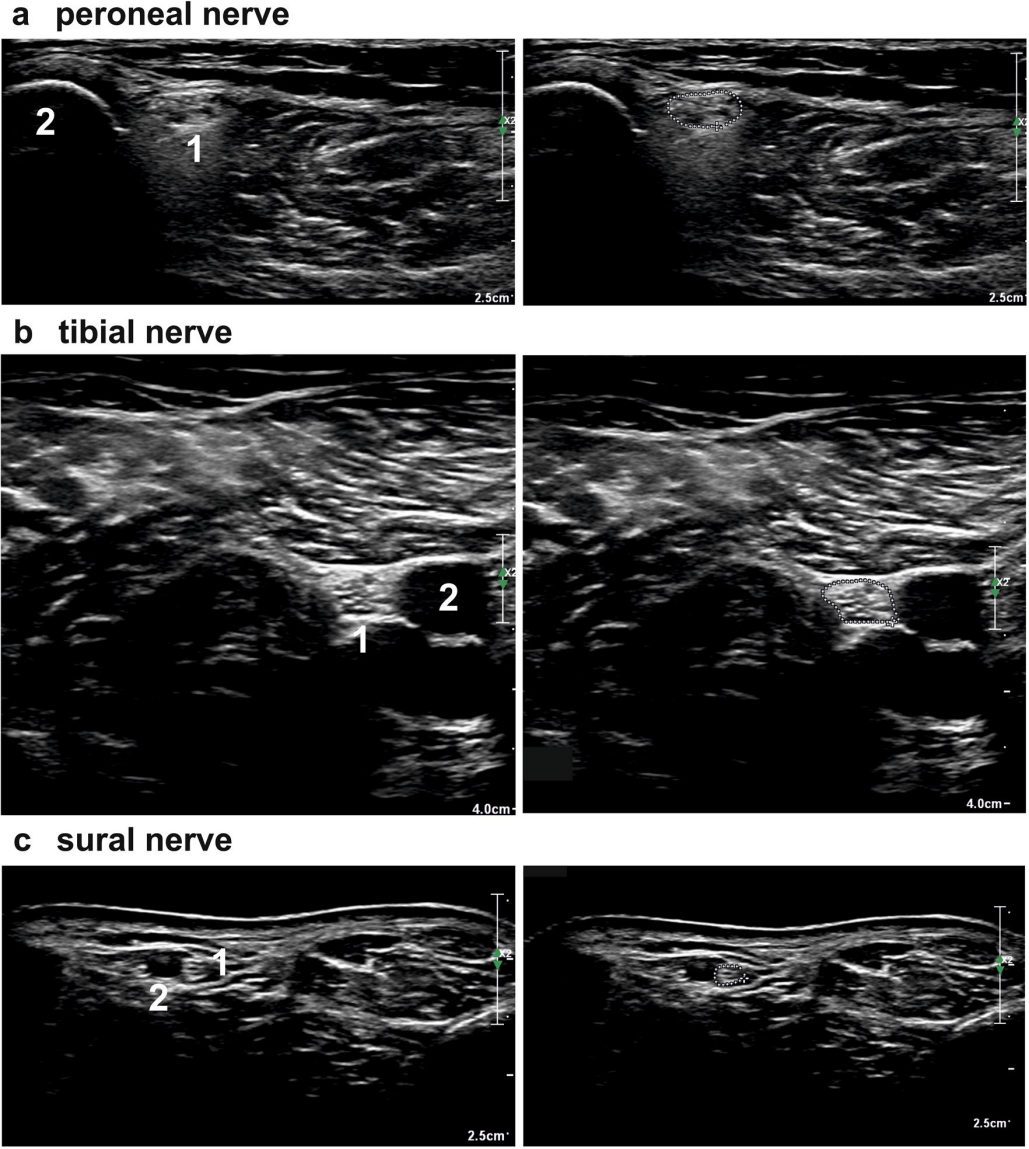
Depicts examples of sectional sonographic images of nerves surrounded by the typical anatomic landmarks. There are two sections provided for each nerve, with and without the marking of the cross sectional area (a: peroneal nerve (1: peroneal nerve; 2: fibula); b: tibial nerve (1: tibial nerve; 2: arteria poplitea); c: sural nerve (1: sural nerve; 2: small saphenous vein).
